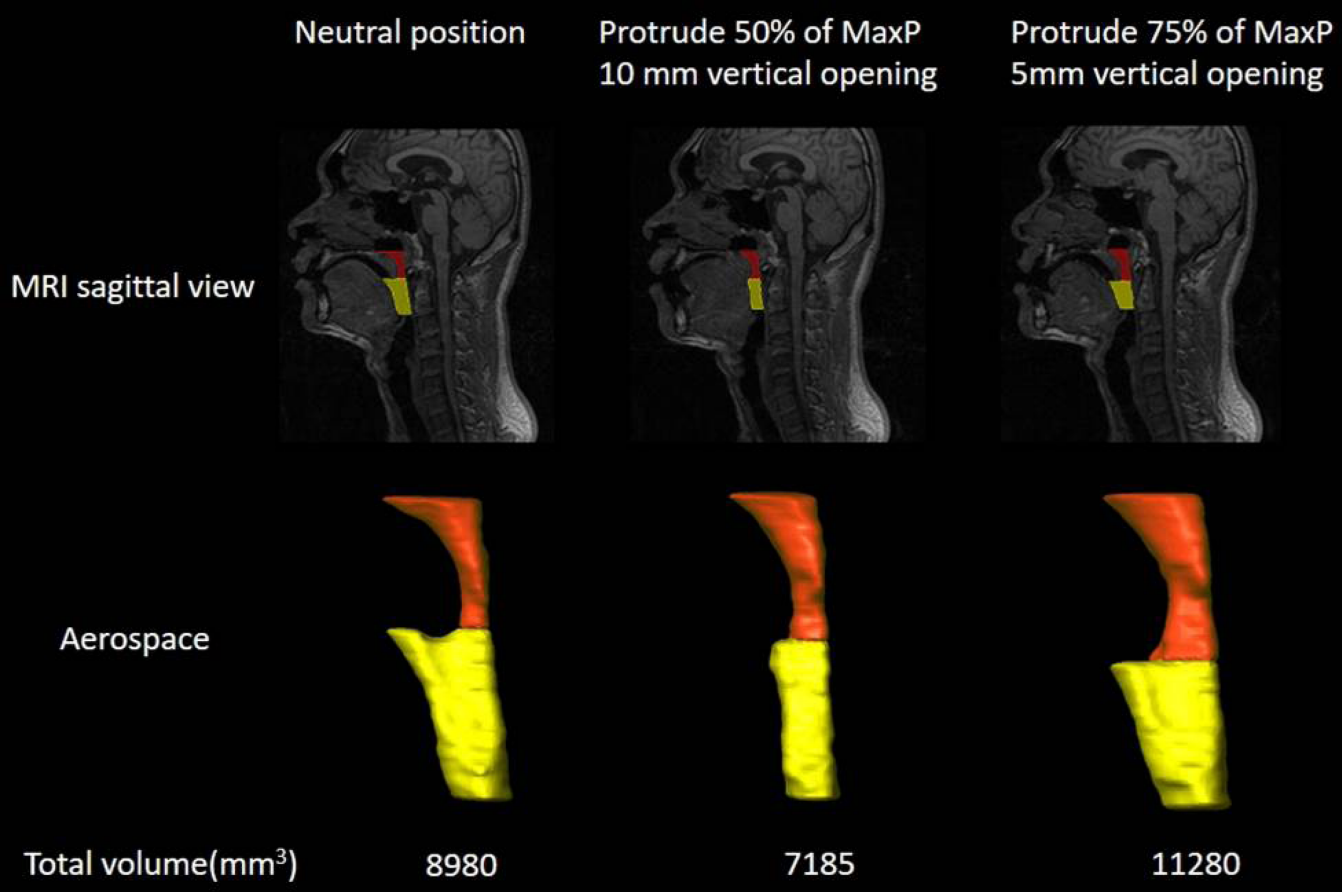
Mid-sagittal MRI demonstrating volumetric reconstruction of airway aerospace at different mandibular advancement and vertical opening in one representative patient with sleep apnea. Oral bite designed for 75% of MaxP with 5mm opening increased the total volume of upper airway more than that in neutral position or that for 50% of MaxP with 10mm opening. The retropalatal space (orange) was defined as the space between the inferior border of the hard palate and the inferior tip of the uvula. The retroglossal space (yellow) was defined as the space between the inferior border of the uvula and the superior tip of the epiglottis. The total volume is the sum of retropalatal and retroglossal volumes.
